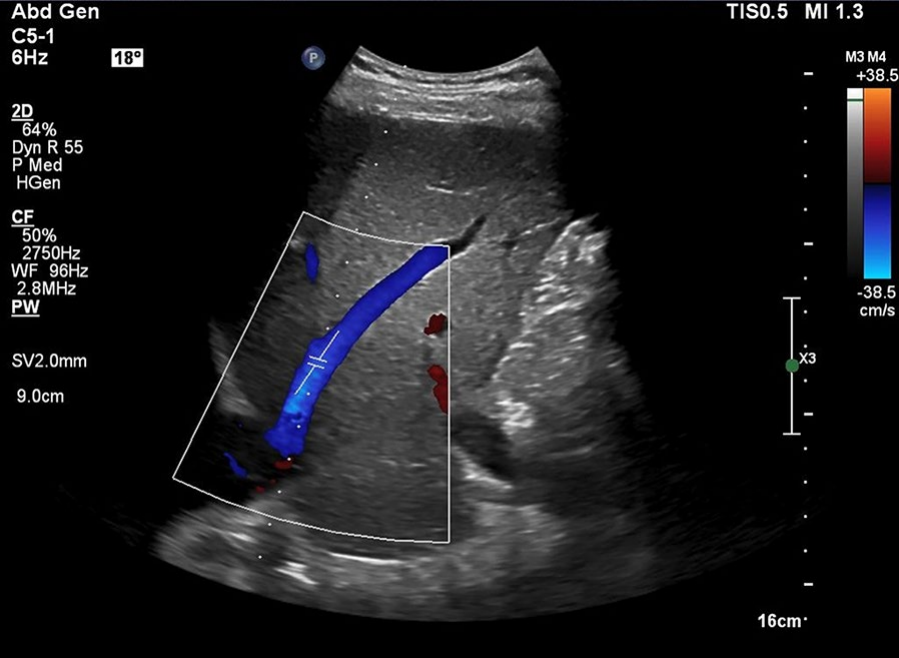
Pulsed wave Doppler mode with the sample volume (Doppler gate) positioned within the HV, approximately 1–2 cm away from its junction with the IVC. IVC, inferior vena cava; HV, hepatic vein
